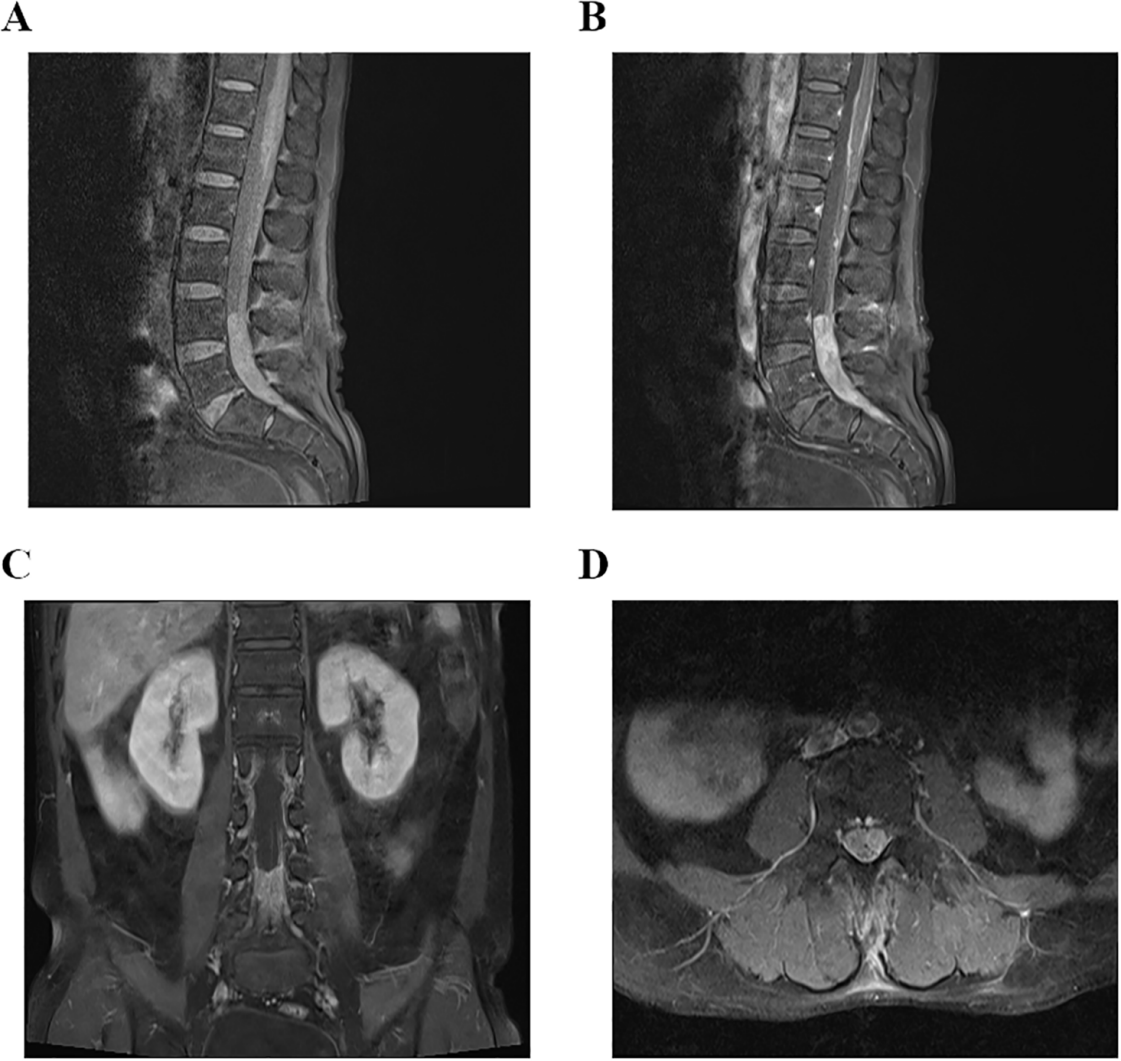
(A–D) MRI examination of lumbar spine on December 15, 2023, indicated that there was an occupying lesion in the L4–S1 intraspinal area, and the size was 1.5 × 1.4 × 9.2 cm.
